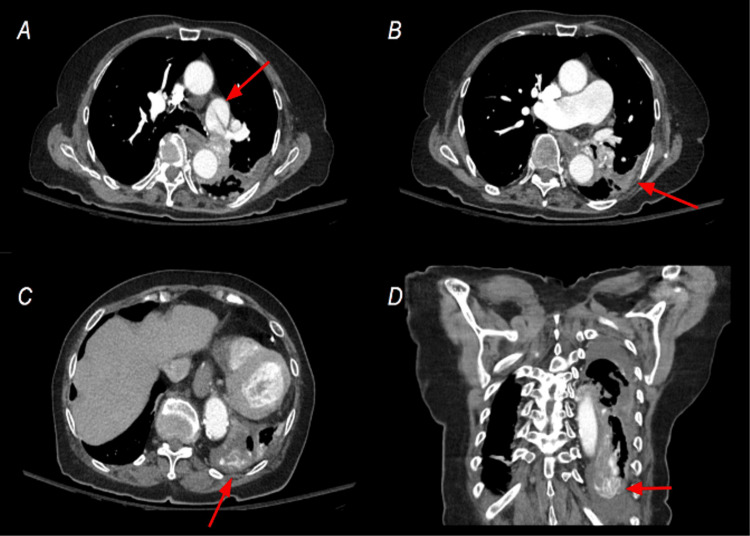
Pulmonary Artery Dissection on Contrast-Enhanced Chest CT A dissection flap within the main pulmonary artery (A) appears to extend into the lobar artery of the left lower lobe, resulting in thrombus and occlusion. Parenchymal disease in the left lower lobe (B) and a relatively dilated right main pulmonary artery can suggest either increased compensatory flow to a non-diseased right lung or underlying pulmonary artery hypertension. Chronicity of this finding was further supported by contrast-filled collateral vessels supplying the inferior portion of the left lower lobe (C,D).
